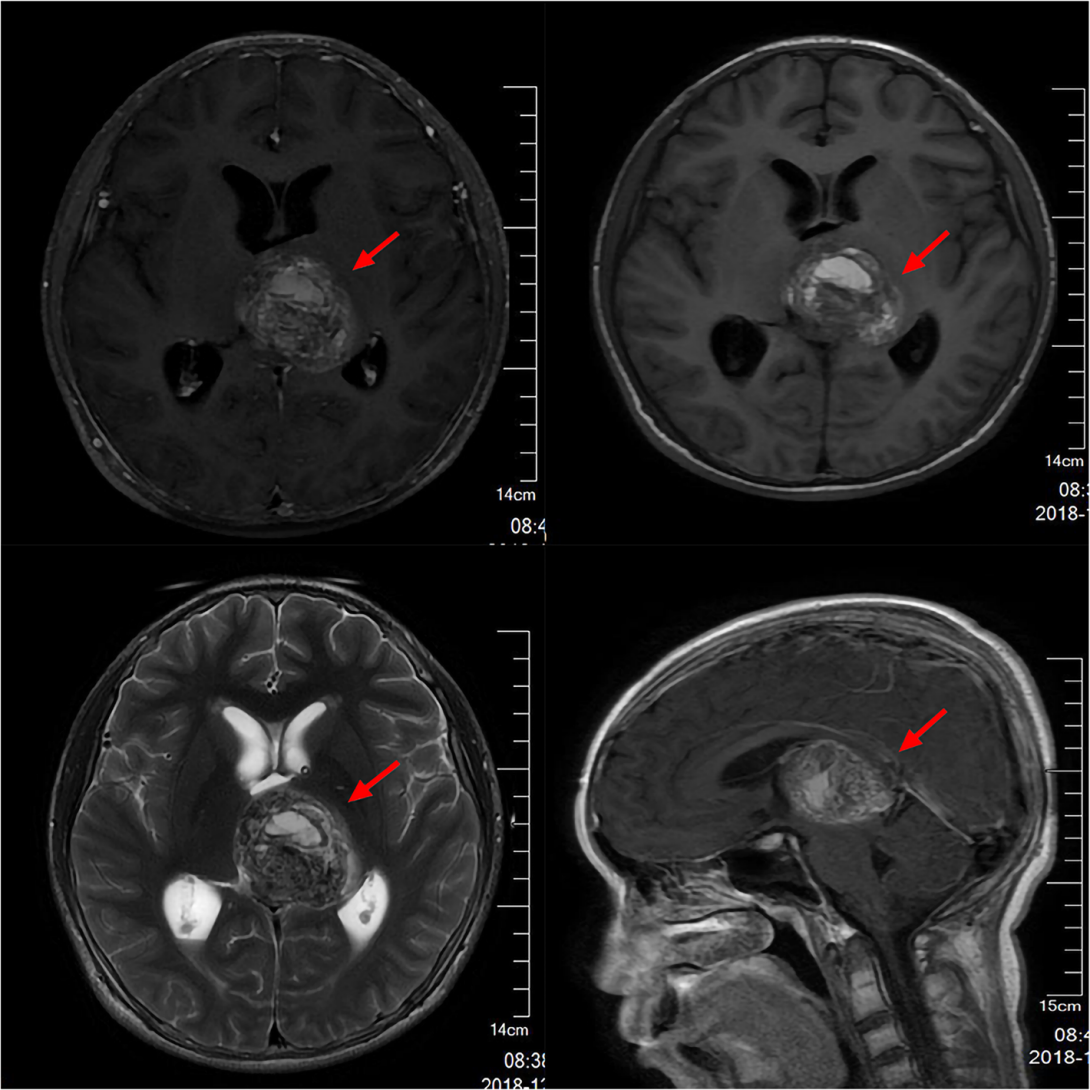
An MRI scan revealed a tumor arising from the pineal region involving the left thalamus with hydrocephalus; the tumor is marked with a red arrow
